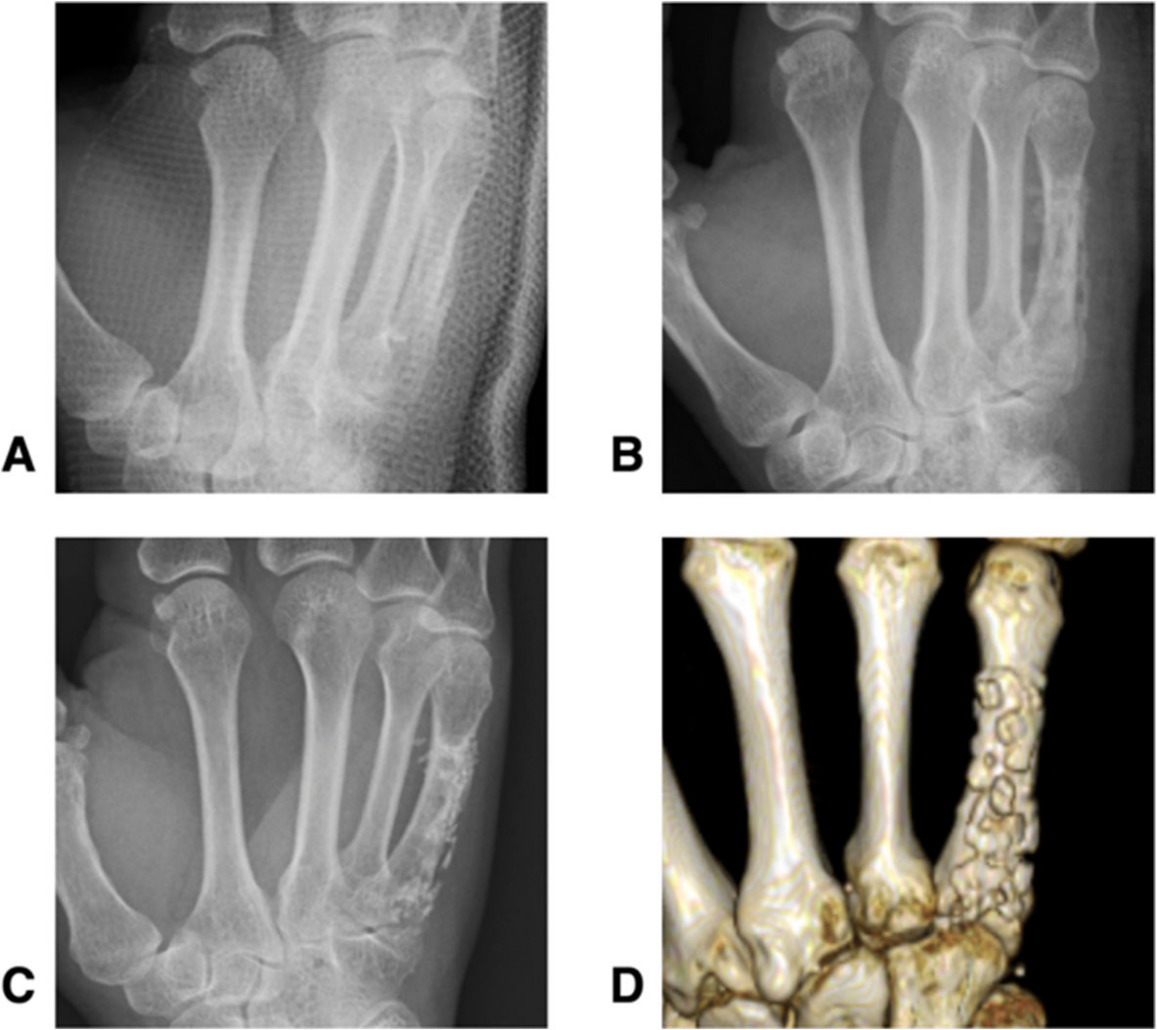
Radiographic images of a 52-year-old female with an oblique metacarpal fracture of the right little finger (Case 5). The a preoperative radiograph, b postoperative radiograph, c final follow-up radiograph (Post 7 years 2 months), and d final follow-up 3D-computed tomography images are shown
